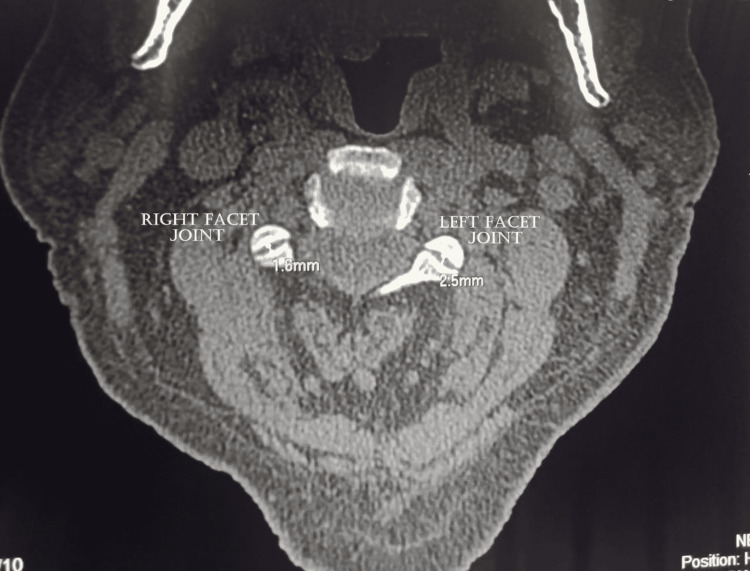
Axial CT image of the cervical spine showing measurements of right and left facet joints.
